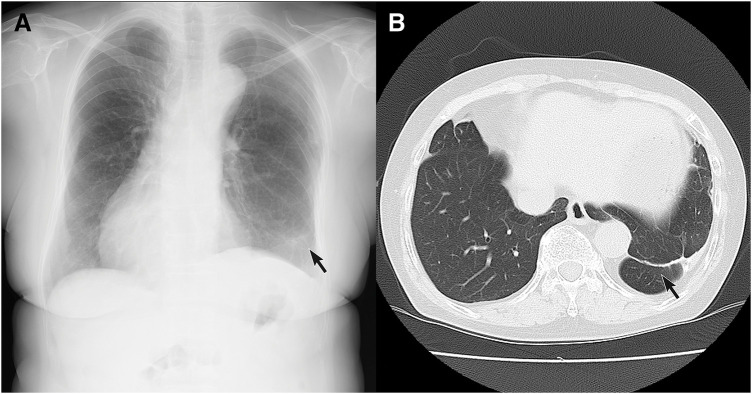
(A) Postoperative chest X-ray shows a staple line in the left S10 region (arrow). (B) Similarly, postoperative chest CT in lung window settings reveals a staple line in the left S10 region (arrow), with no evidence of abnormal pulmonary vascular engorgement.
